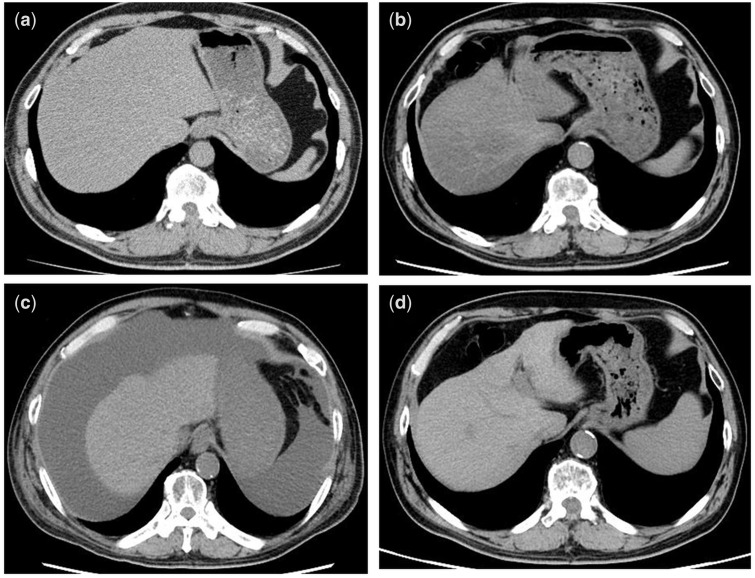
CT scan of a patient with MTX-associated reversible liver atrophy in a patient with RA (a) Seven years before hospitalization. (b) Three years before hospitalization. (c) At the time of hospitalization. (d) Ten months after MTX discontinuation.
